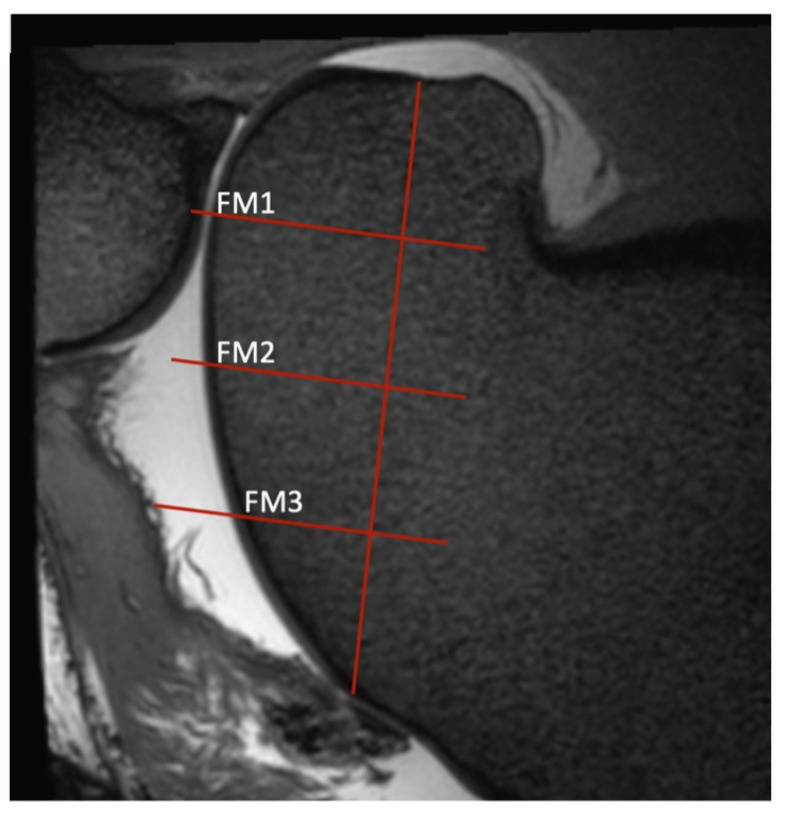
Sagittal view of a T2-weighted image of the medial trochlear ridge of a stifle, as determined by the procedure described in Figure 1. Three regions of interest (ROIs) were defined. In the sagittal view, a line was drawn from the notch where the trochlea ends and the condyle starts up to where the trochlear cartilage ends. This stretch was then quartered, resulting in three lines perpendicular to the original line. Where these lines intersect with the articular cartilage, ROIs (FM1, FM2, and FM3) were determined and the cartilage thickness was measured. The same process was repeated for the lateral dorsal view in the respective sagittal planes. For the lateral measurements, the middle of the origin of the lateral digital extensor tendon was used as the starting point of the vertical line and the proximal edge of the cartilage as the end. For the trochlear groove, the distal and proximal cartilage edges were used as starting and end points. As on the medial trochlear ridge, these two lines through the lateral trochlear ridge and the central groove were quartered with lines perpendicular to the first, resulting in three ROIs each.
